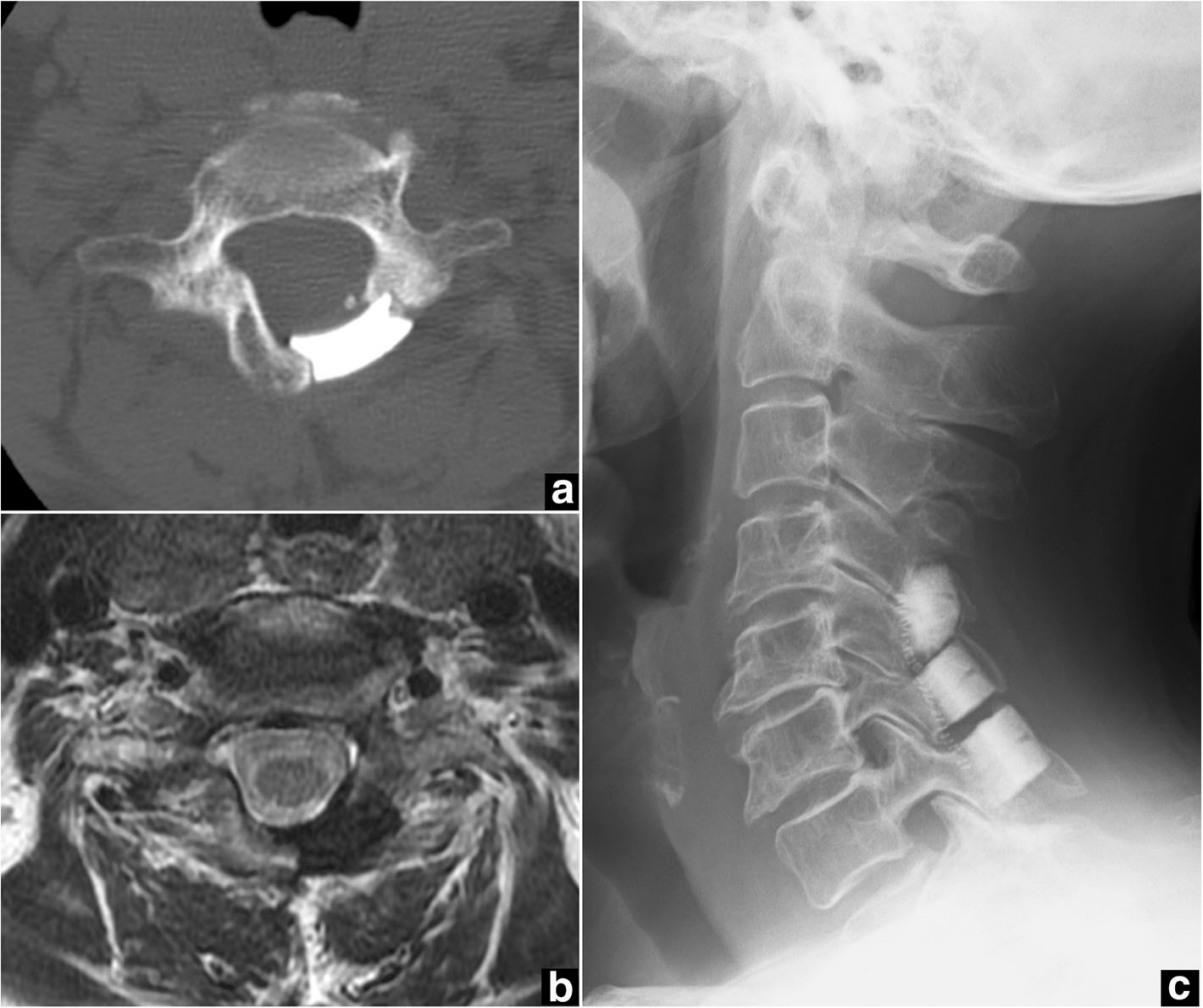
Case 2. Postoperative computed tomography (CT), magnetic resonance imaging (MRI), and plain X-ray of the cervical spine. a Axial CT at the C5 level 1 year after surgery demonstrates a newly created spinal canal with HA spacers bonded to host bone. b Axial MRI at the C5 level obtained 5 years after surgery shows no tumor recurrence with good visualization inside the spinal canal. c Lateral plain X-ray obtained 6 years after surgery shows normal sagittal alignment
